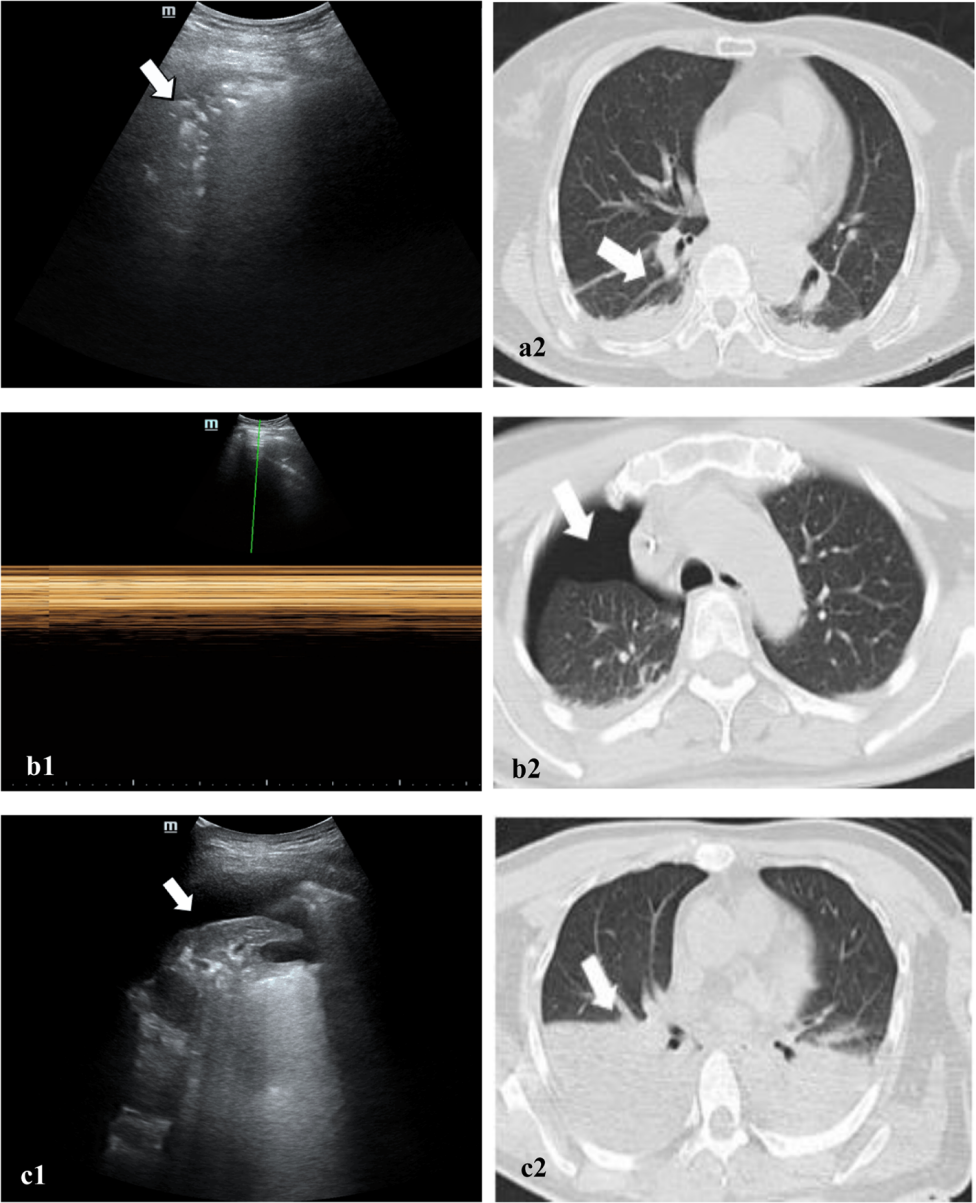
Typical pulmonary pathologies on both LUS and thoracic CT in the same regions. (a1) Typical LUS signs of atelectasis in the dorsal quadrant of the lung presented as tissue-like patterns (left, white arrow), (a2) CT signs of corresponding regions presented as a crescent shape (right, white arrow) (b1) Typical LUS on M-mode of pneumothorax in the anterior quadrant of the lung presented as a bar code sign, (b1) CT signs of corresponding regions presented as very-low-density gas window (right, white arrow). (c1) Typical LUS of pleural effusion in the dorsal quadrant of the lung presented as anechoic area (left, white arrow), (c2) CT signs of corresponding regions presented as a half-moon (right, white arrow). Abbreviations: LUS, lung ultrasound; CT, computed tomography.
